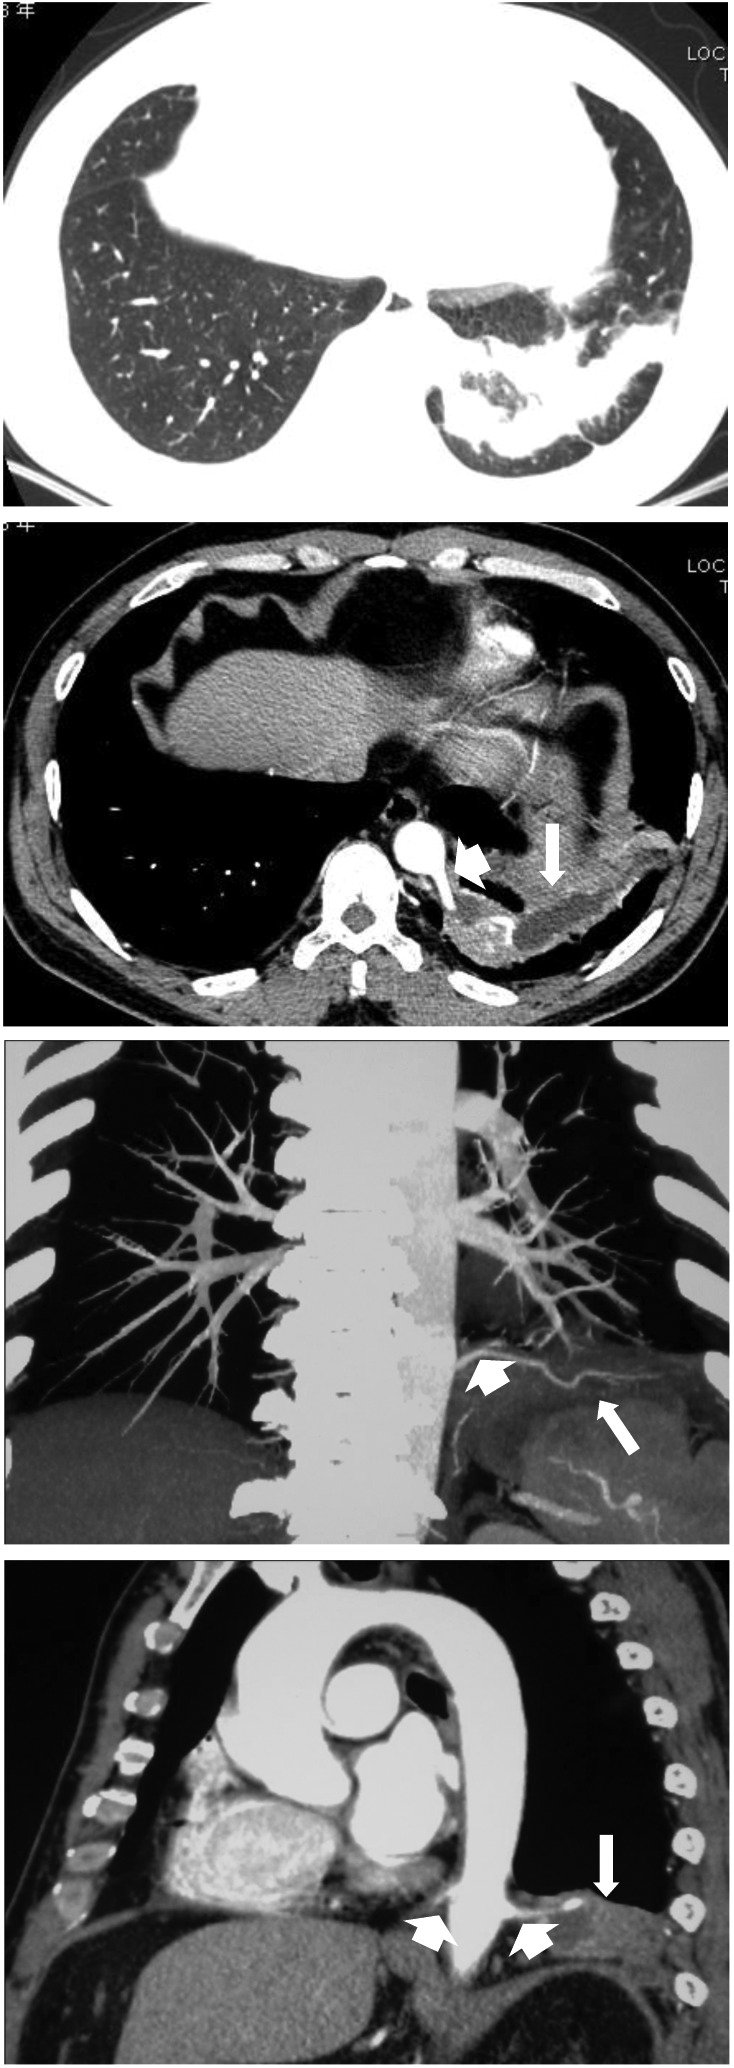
Chest high-resolution computed tomography scanning and 3D image reconstruction showed a mass in the left lower lobe and two anomalous arteries (short arrow) arising from the descending thoracic aorta and extending into the sequestered lung (long arrow).
